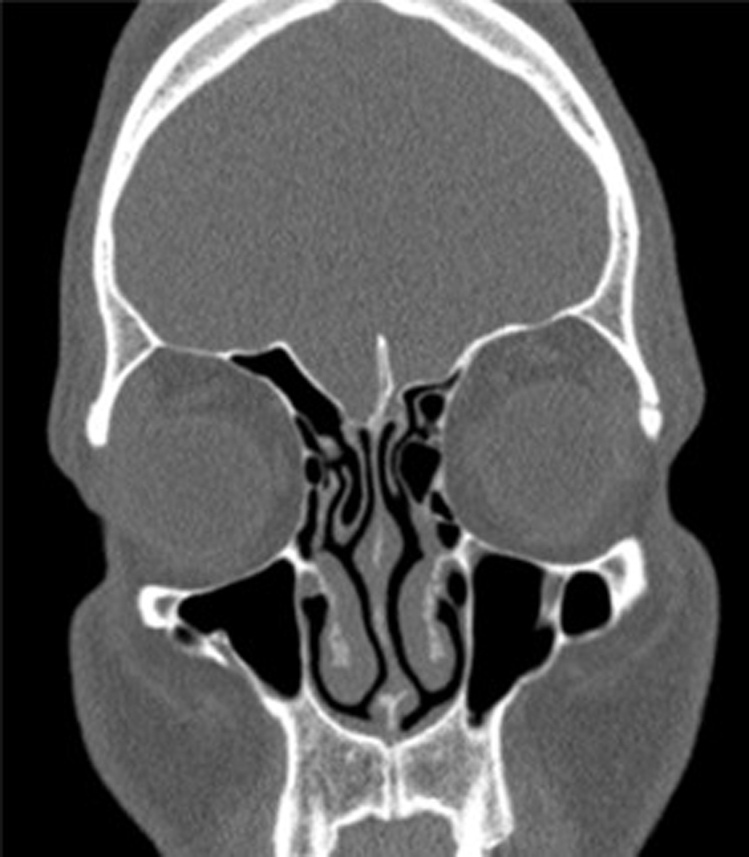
Etmoid roof asymmetry and concha bullosa. Unilateral concha bullosa and ethmoid roof asymmetry are evident. Coronal section the paranasal sinus CT image is the section where the infraorbital nerve was first seen, and all measurements were taken from this section.
